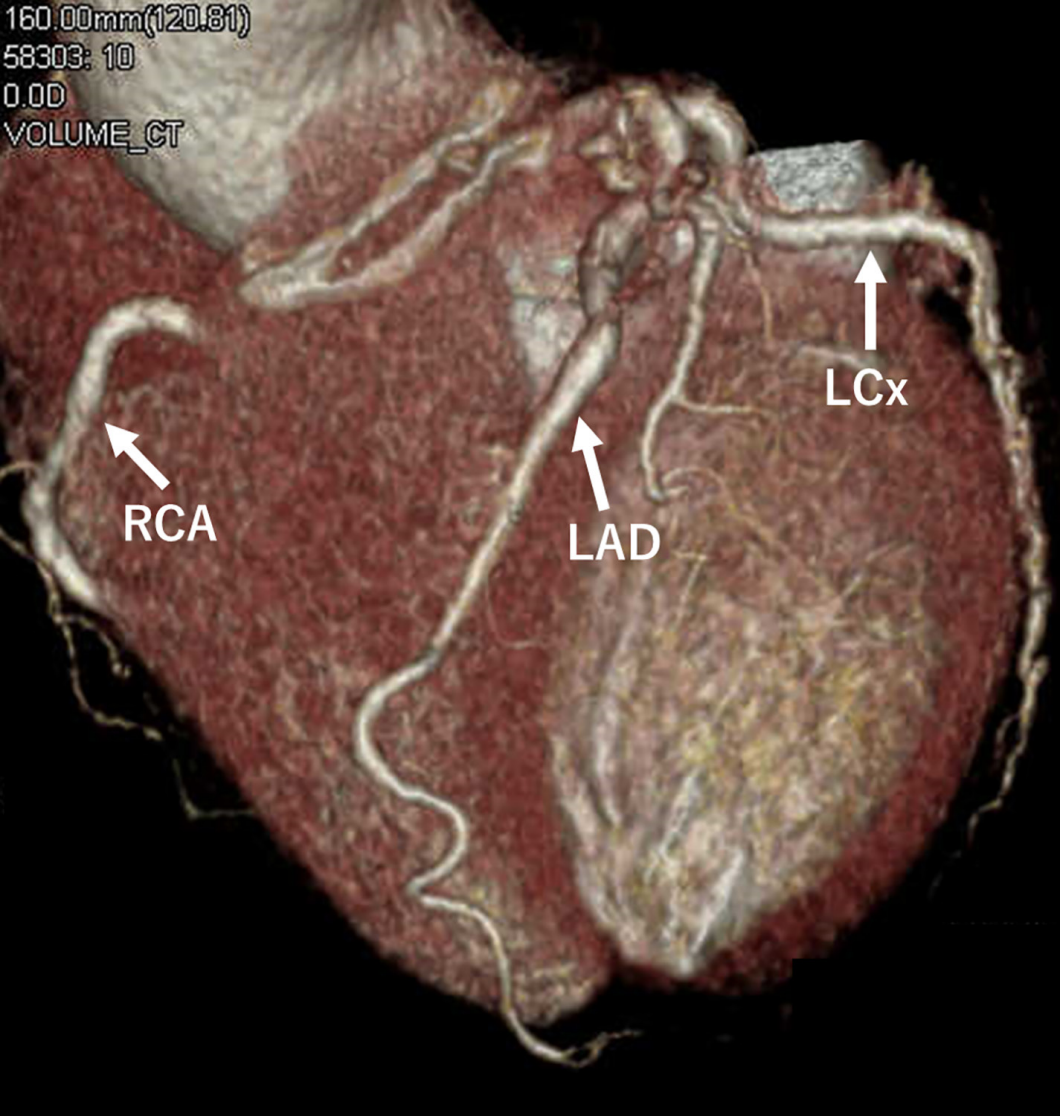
Postoperative contrast-enhanced CT findings. Postoperative contrast-enhanced CT reveals no residual coronary artery aneurysms. LAD, left anterior descending artery; LCx, left circumflex artery; RCA, right coronary artery
